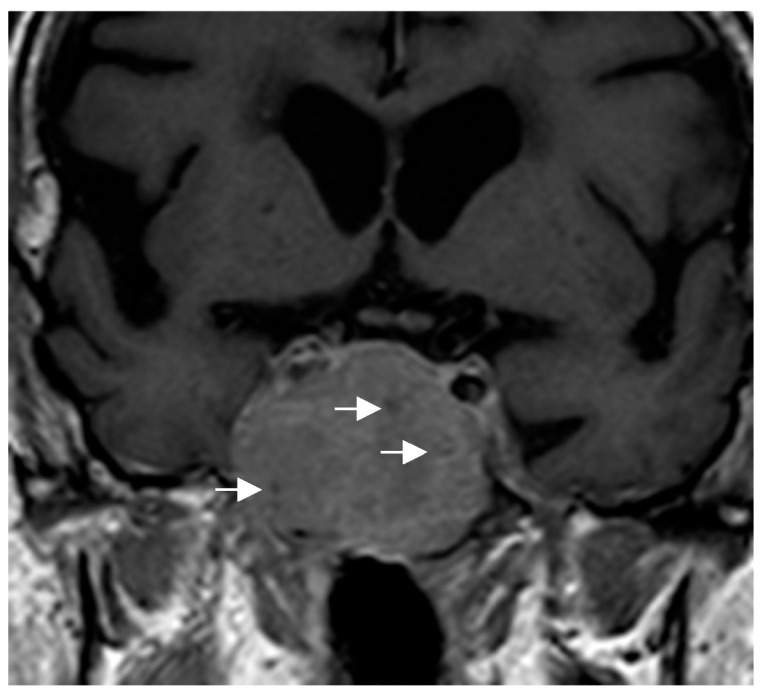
Coronal T1-weighted image showing an intra-suprasellar spindle cell oncocytoma (SCO). Multiple hypointense millimetric foci (white arrows) are seen in the lesion.
